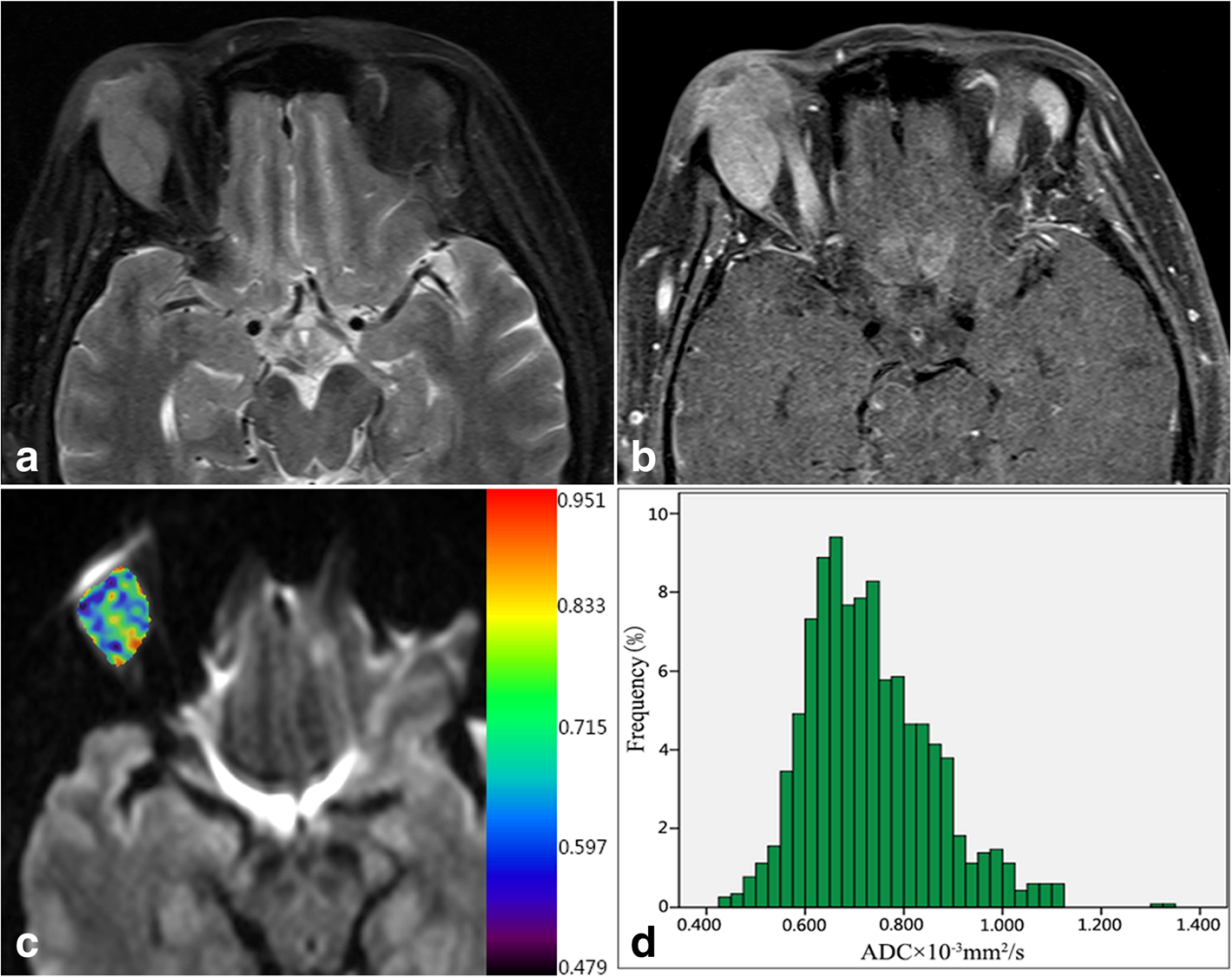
A female patient with idiopathic orbital inflammatory pseudotumor. Axial fat-saturated T2W image a shows a well-defined isointense mass in lacrimal fossa. Axial contrast enhanced T1W image b demonstrates significant enhancement, similar to that of extraocular muscles. Pixel-by-pixel colored ADC map c was obtained and then embedded with the axial DWI. The corresponding ADC histogram from VOI d a relatively flat peak and a slight tail on the right. Whole-tumor ADC_mean_ was 0.730 × 10^− 3^ mm^2^/s, ADC_10_ was 0.590 × 10^− 3^ mm^2^/s, skewness was 0.73, and kurtosis was 0.80
